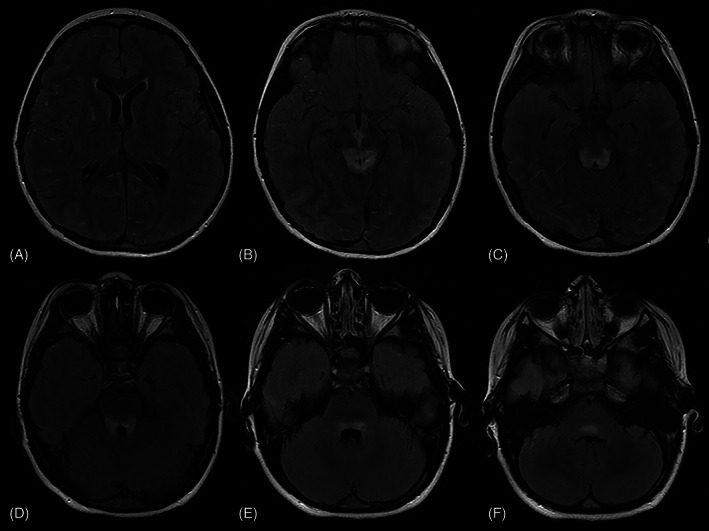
Magnetic resonance imaging of the brain on day 2 of admission showing T2/fluid‐attenuated inversion recovery (FLAIR) sequences. Imaging obtained at 9 years and 7 months of age. Axial T2/FLAIR sequences of the brain demonstrate T2/FLAIR hyperintensity within the right globus pallidus (A), dorsal mid brain and periaqueductal grey matter (B, C) and within the dorsal pons (D–F).
